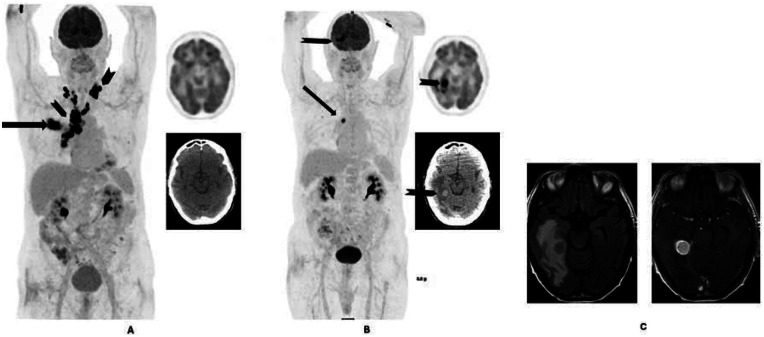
80-year-old male with lung cancer. The staging 18F-FDG PET/CT (A) from vertex to mid-thigh shows metabolically active right lung primary (arrow) with hilar, bilateral mediastinal, and supraclavicular nodal spread (arrowheads). Follow-up 18F-FDG PET/CT (B) 5 months after treatment with four cycles of carboplatin, premetrexed, and pembrolizumab shows a dramatic response in the primary disease sites and the nodes (arrow). However, there is a new focus of uptake in the brain (arrowhead) in keeping with brain metastasis. This was confirmed on MRI (C), which shows an enhancing right temporal lobe lesion with perilesional edema. Brain metastasis was not clinically suspected at the time of the follow-up PET/CT.
